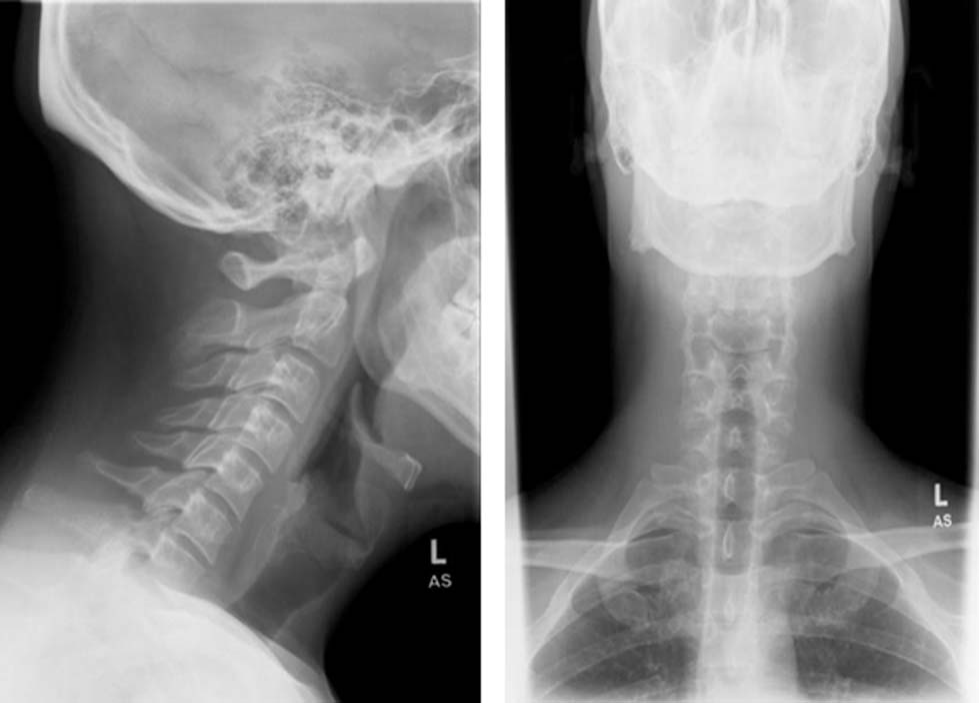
Initial X-rays of the cervical spine (AP and lateral views) demonstrating no obvious spinal pathology.
